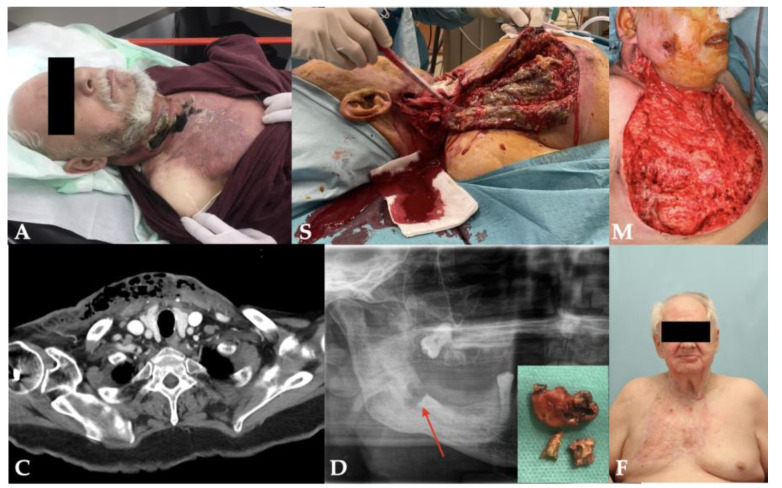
Clinical course and radiographs of patient No. 3: A: admission; S: surgery; M: maximal extent; F: final result; C: CT scan; D: dental focus (empty alveolus and decayed tooth 48).
